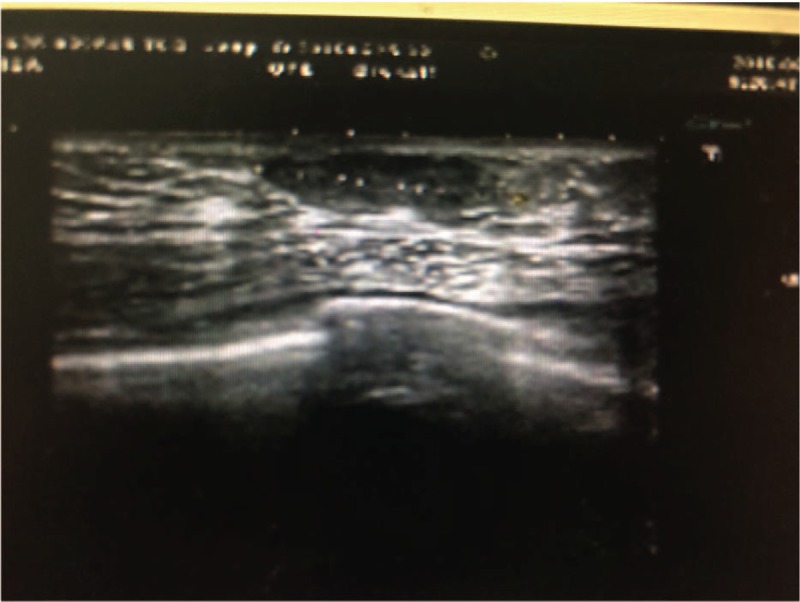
Ultrasound image, showing a well-encapsulated lesion.
